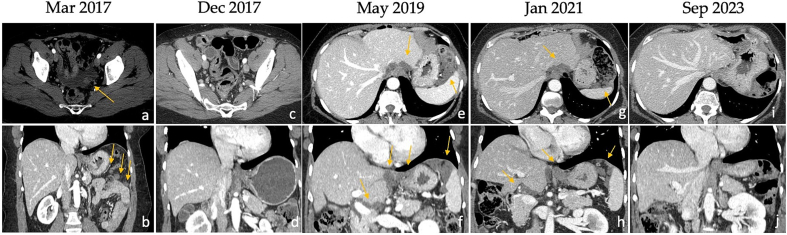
CT scan: In March 2017 the first relapse of the disease was assessed at our radiology department. At CT, on the axial plane (a), a small amount of free fluid and peritoneal nodules are visible, quite evident also along the left parietocolic gutter in the coronal plane (b). After CRS and HIPEC (April 2017) the CT in December 2017 (c, d) was completely negative. In May 2019 the patient had a second relapse and peritoneal irregular thickening consisting of confluent nodules is detectable near the spleen, under the left diaphragm and along the hepatic capsule (e, f). In January 2021 only a small reduction was visible (g, h) but it was considered enough to attempt a new treatment by CRS and HIPEC. At the most recent follow-up CT scan, in September 2023, there are no signs of relapse.
